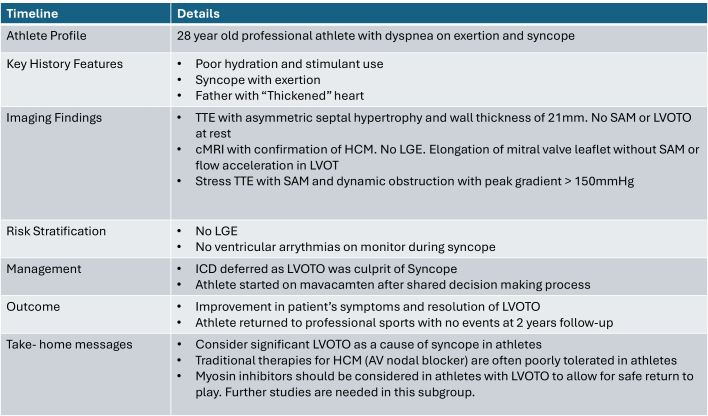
Athlete's Case Presentation A 28-year-old professional athlete with exertional syncope was found to have asymmetric septal hypertrophy without resting obstruction. Stress echocardiography revealed severe dynamic left ventricular outflow tract obstruction (>150 mm Hg). After intolerance to beta-blockers, mavacamten was initiated, with resolution of obstruction and symptoms, allowing return to professional sports without events at 2-year follow-up. AV = atrioventricular; cMRI = cardiac magnetic resonance imaging; HCM = hypertrophic cardiomyopathy; LVOT = left ventricular outflow tract; LVOTO = left ventricular outflow tract obstruction; ICD = implantable cardioverter-defibrillator; LGE = late gadolinium enhancement; SAM = systolic anterior motion; TTE = transthoracic echocardiography.
